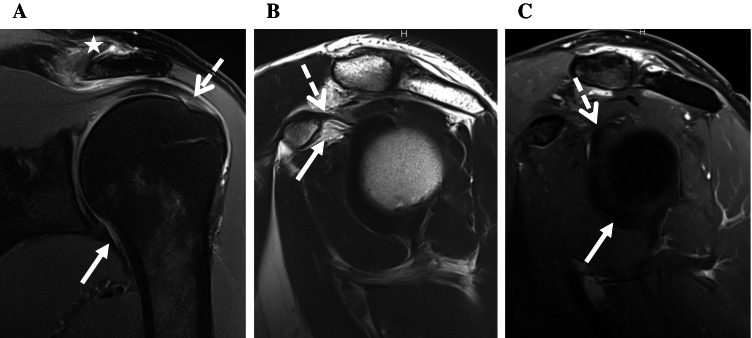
MR images from the left shoulder of a 49-year-old male with painful left shoulder but neither clinical nor MR imaging signs of ACS. A Fat-saturated oblique coronal PDw image shows a normal capsule of the axillary recess (arrow) without thickening or signal increase. There is tendinosis of the supraspinatus tendon (dotted arrow) and osteoarthritis of the acromioclavicular joint (star). B T2w oblique sagittal image demonstrates normal subcoracoid fat (arrow) as well as normal CHL (dotted arrow). C T1w fat-saturated oblique sagittal image after IV contrast shows no contrast enhancement of the capsule in the rotator interval (dotted arrow) and the axillary recess (arrow)
